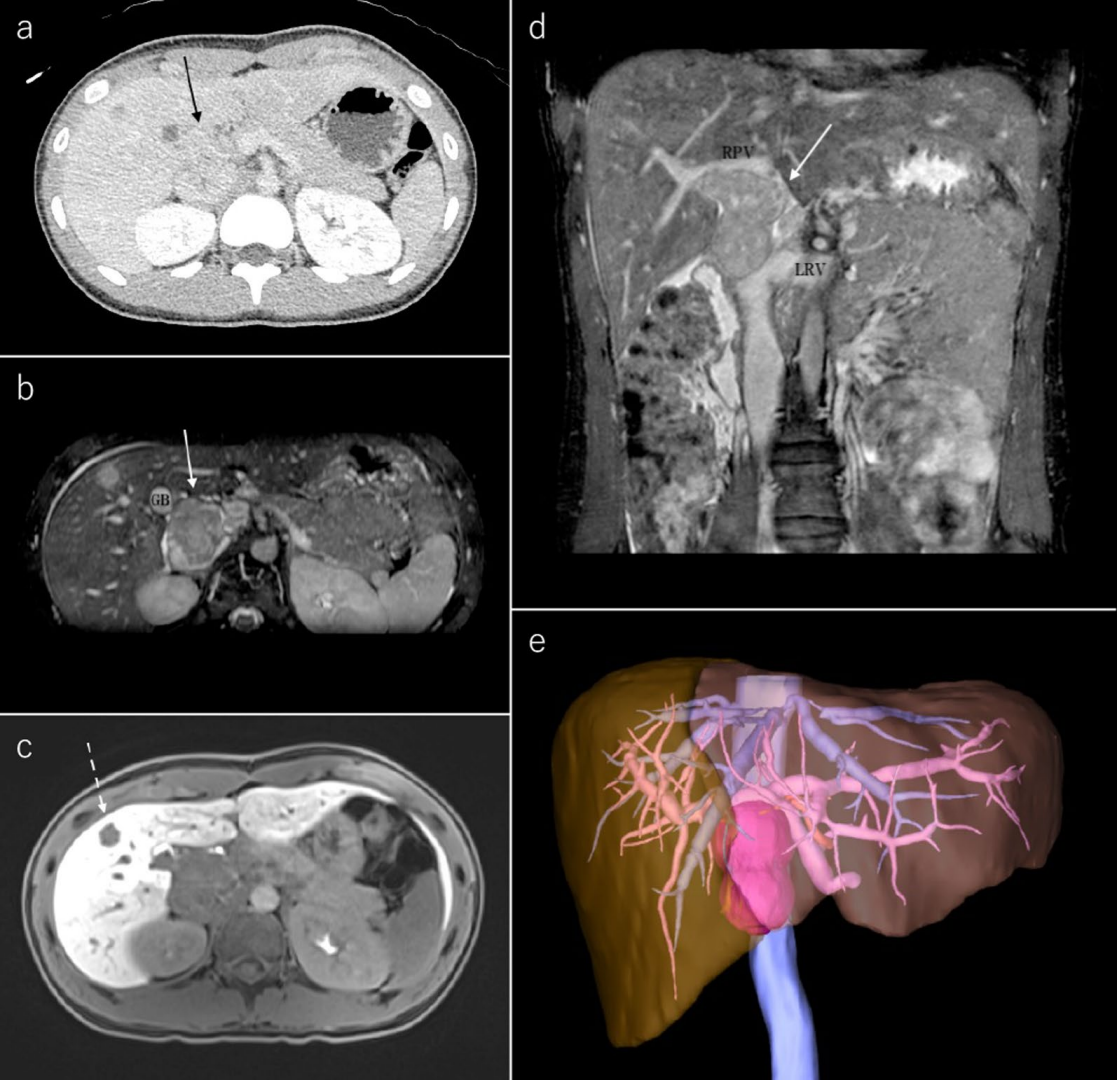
a–d Contrast-enhanced abdominal CT (a) and MRI (b–d) showed a 6 × 4 × 5 cm mass (solid arrow) protruding into the lumen of the IVC. The mass was located slightly superior to the renal vein confluence, ventrally compressing the hepatic hilum, and the right portal vein was stenotic. The MRI showed areas of decreased Gd-EOB–DTPA uptake at S1 (5 mm × 1), S5 (15 mm × 1, dotted arrow), and S6 (5 mm × 1) in the hepatocellular phase (c) e Three-dimensional reconstructed image. *GB* gall bladder, *RPV* right portal vein, *LRV* left renal vein, *CT* computed tomography, *MRI* magnetic resonance imaging
